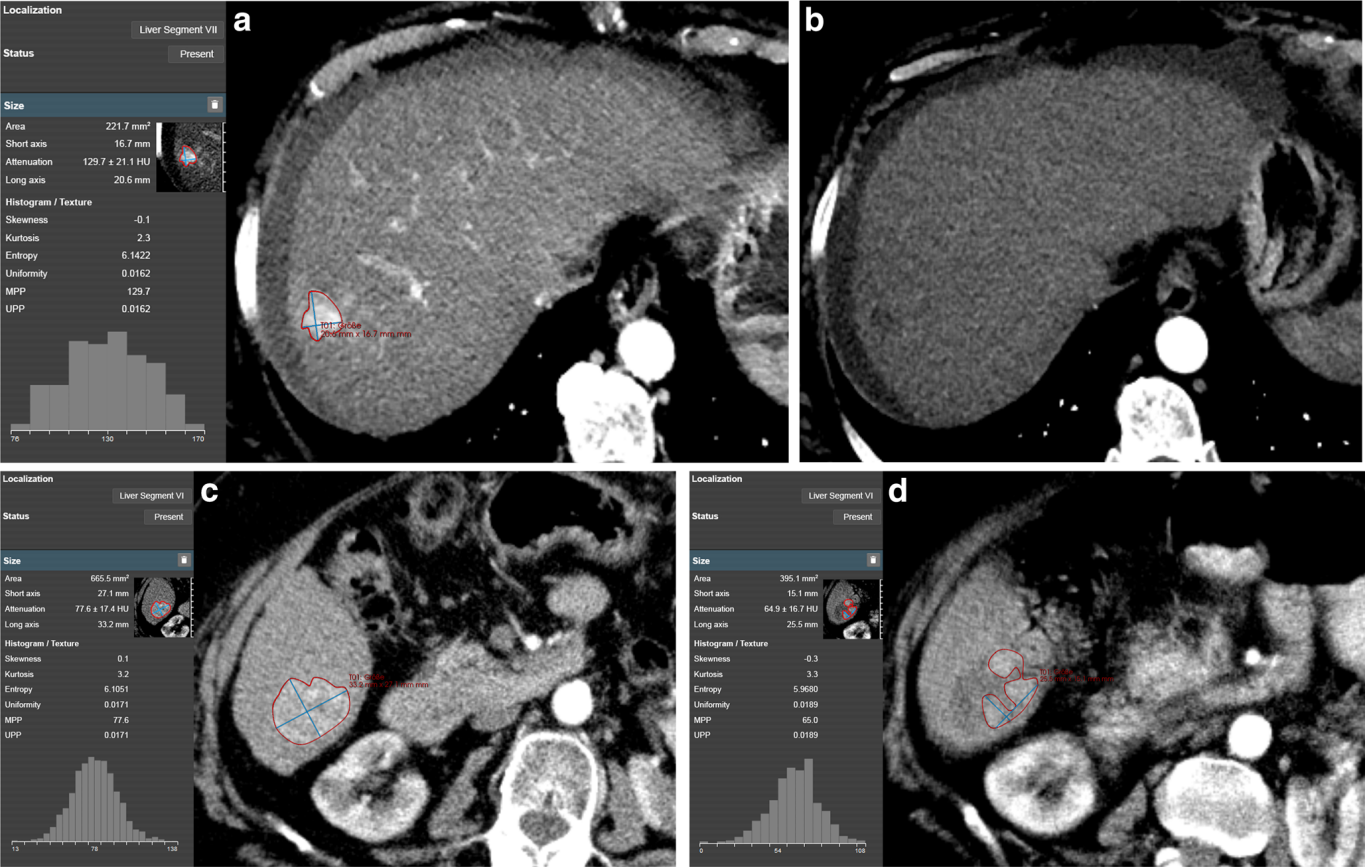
a Screenshot of a manually segmented HCC lesion according to mRECIST criteria on baseline arterial phase imaging in mint Lesion™ 3.0 software with automatic generation of gray-level histograms and extraction of CT texture parameters. Favorable lesion size and parameters from CT texture analysis (especially mean of positive pixels (MPP) and uniformity) render this HCC lesion likely to respond completely to transcatheter arterial chemoembolization (TACE) treatment. b Four weeks after TACE treatment, the HCC shows no enhancement in arterial phase (thus, no viability) resulting in complete response as per mRECIST criteria. c Another manually segmented HCC lesion at baseline imaging. Unfavorable lesion size, surrounding liver parenchyma (cirrhotic), and parameters from CT texture analysis (especially MPP and uniformity) at baseline imaging result in a low likelihood of complete response to TACE treatment. d Follow-up imaging 4 weeks after TACE treatment shows substantial residual arterial enhancement (= viable portions of the tumor), resulting in partial response as per mRECIST criteria. The patient subsequently underwent repeated TACE, and the post-treatment CT images were used as new baseline
